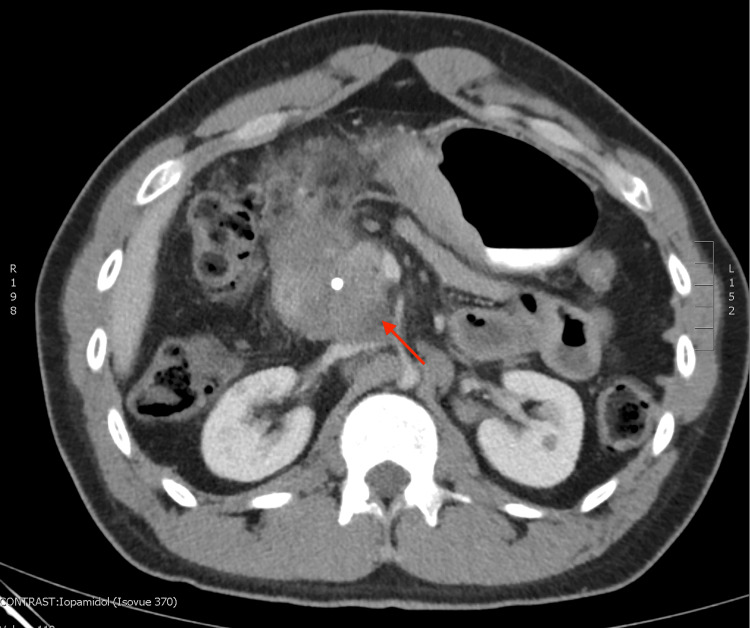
Abdominal computed tomography (axial view) with oral and IV contrast re-demonstrating homogeneous mass, 5.6 cm in the longest dimension, in the region of the pancreatic head circumferentially surrounding the common bile duct stent.
